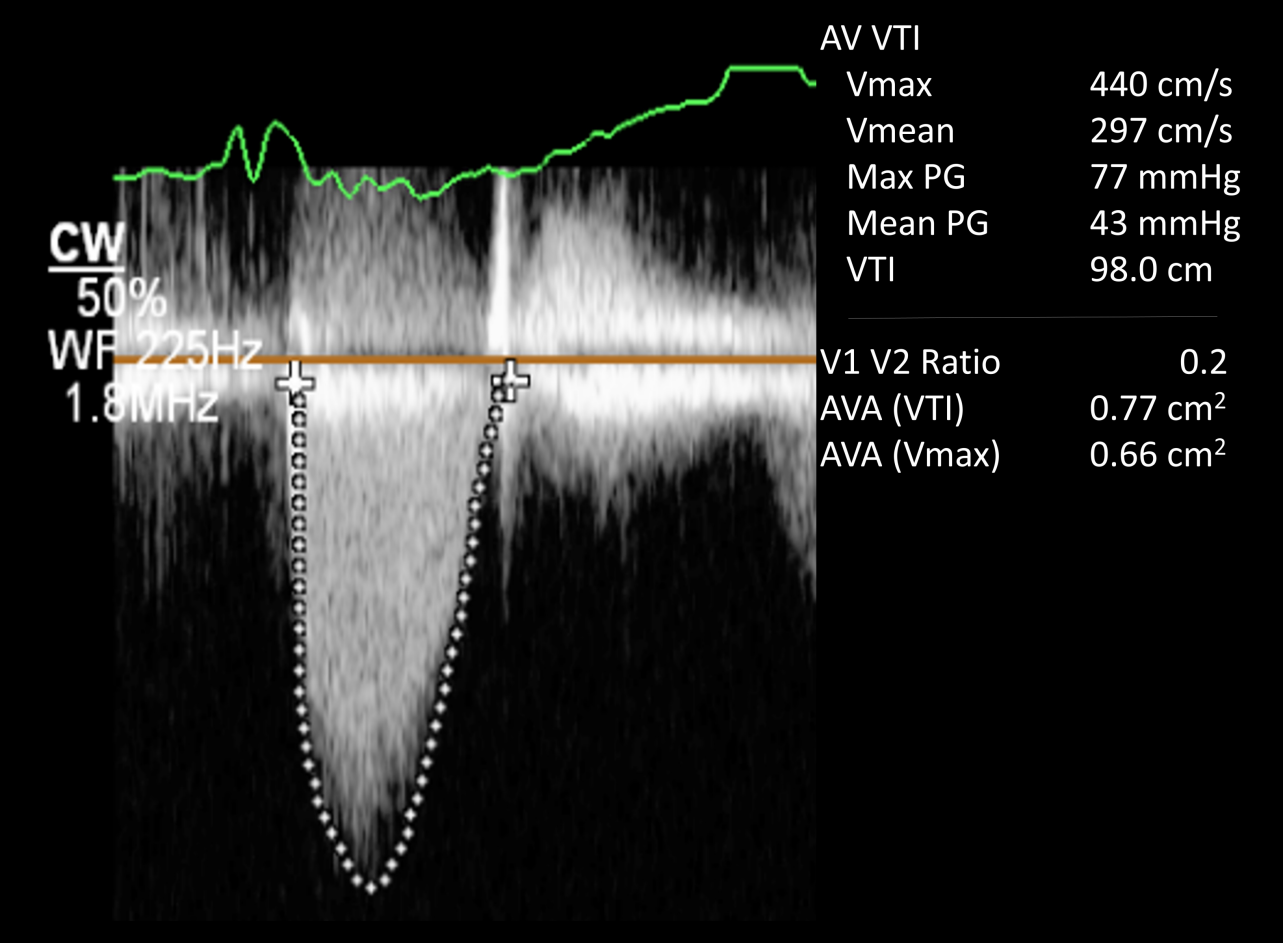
Doppler echocardiogram showing elevated peak and mean gradients across the aortic valve at 77 mm Hg and 43 mm Hg respectively
